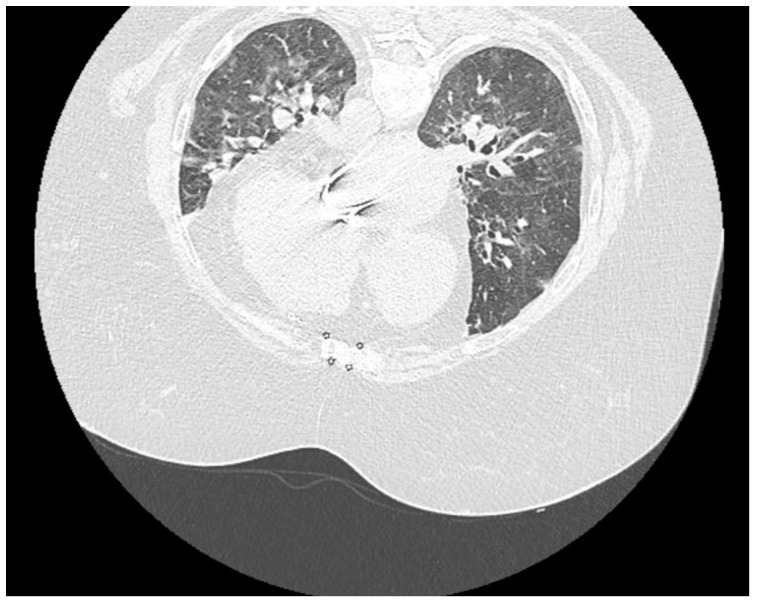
Native thoraco-pulmonary CT of the patient, revealing a viral SARS-CoV-2 pneumonia with a degree of damage around 50–60% and with unsystematized central and peripheral pulmonary infiltrates and ground-glass opacities.
